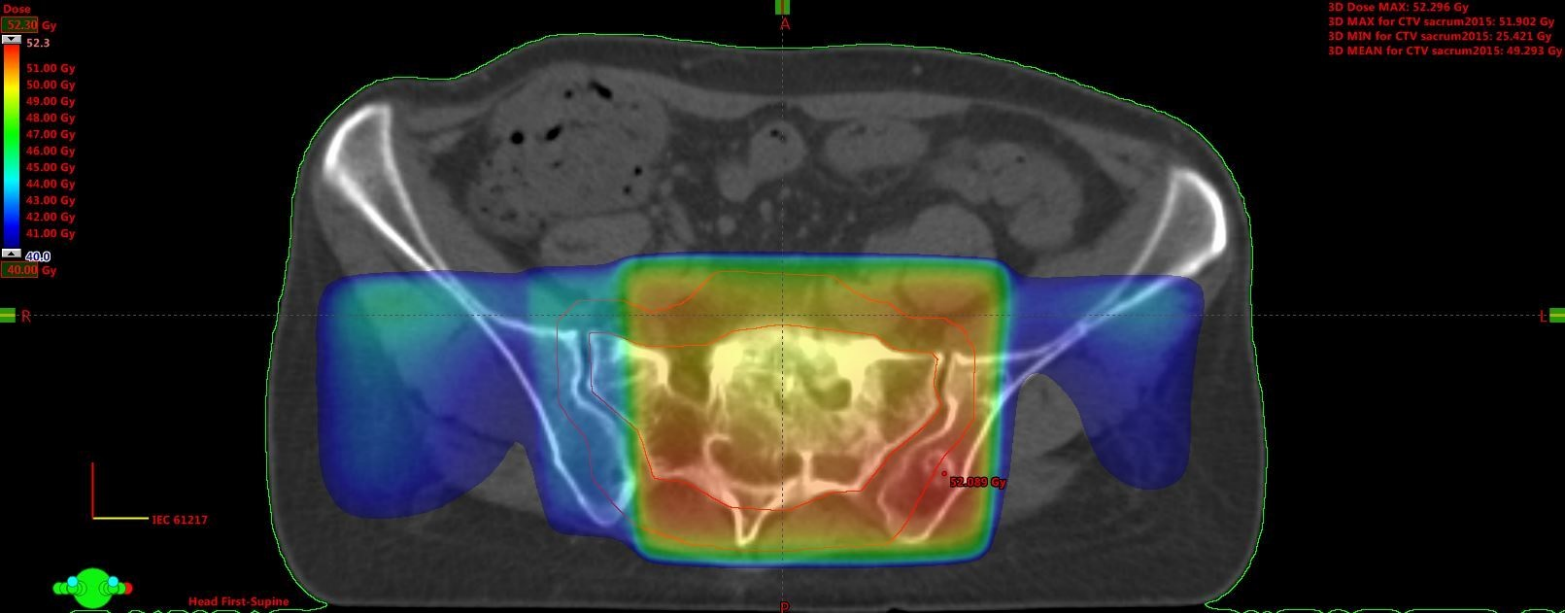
Dose distribution from the co-registered second and third course combined Axial computed tomography (CT) scan, doses >40 Gy are displayed, the maximum dose was 52 Gy. The bowels are located outside the 40-Gy isodose.
